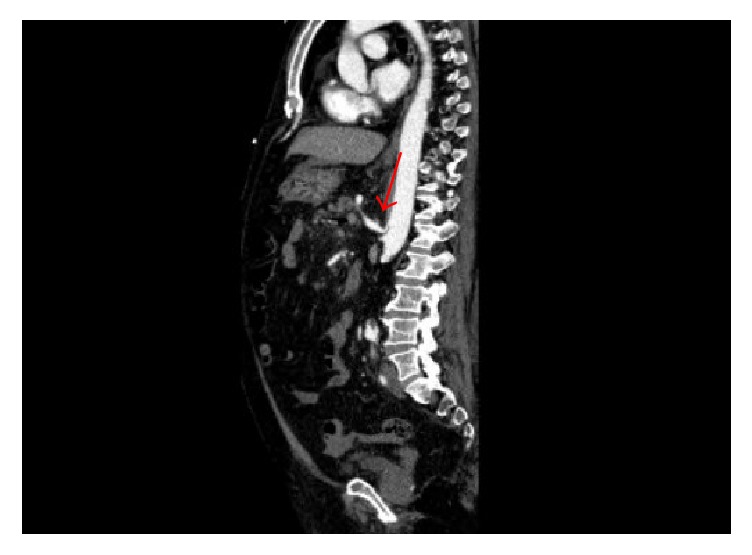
Evidence of celiac trunk stenosis*: Sagittal reconstruction of contrast-enhanced CT scan of abdomen revealing the evidence of stenosis at the take-off of the celiac trunk (Arrow).
